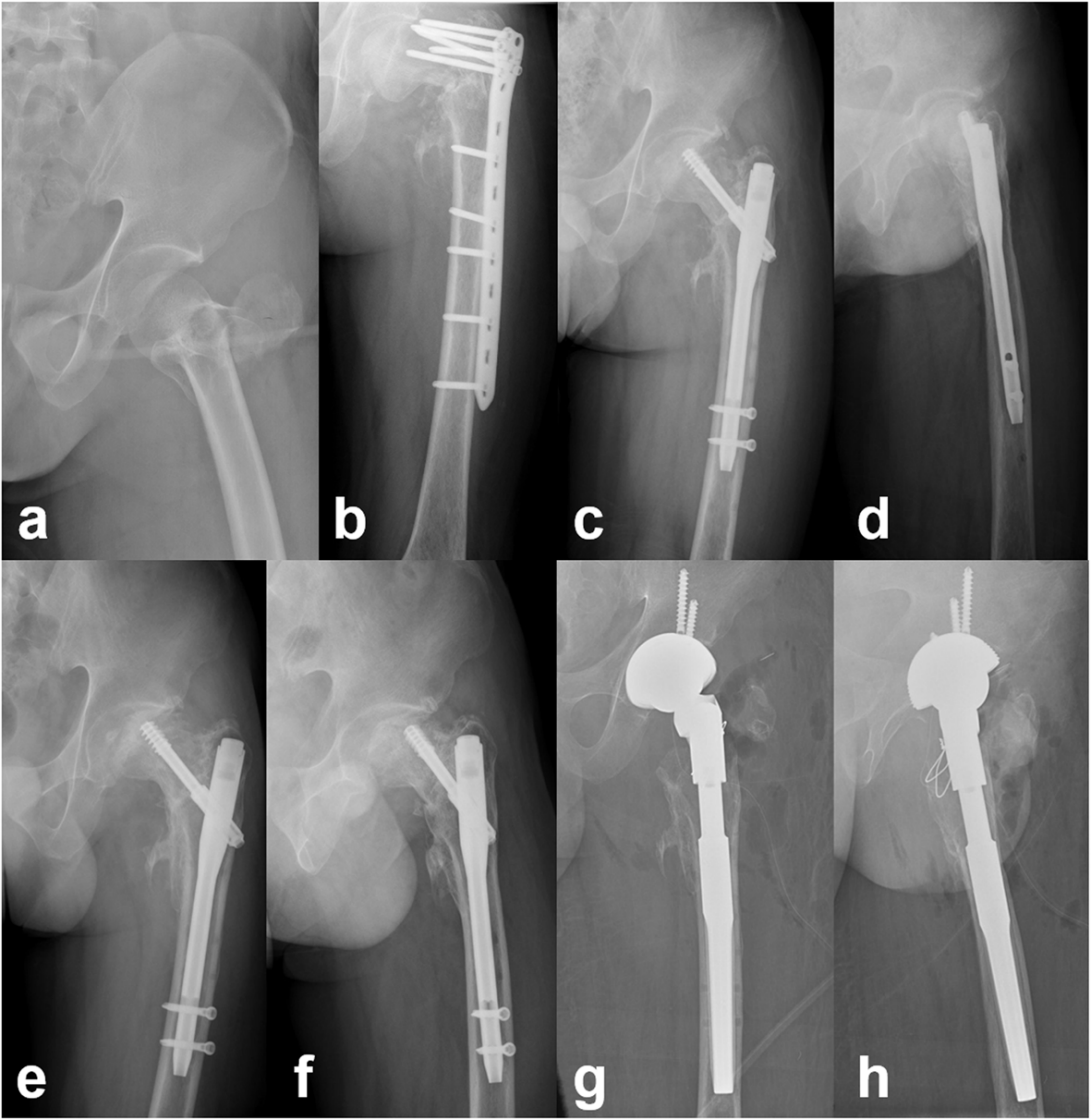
A 42-year-old man with left trochanteric fracture (AO/OTA A3) initially fixed by inverted less invasive stabilization system plate. a The X-ray of original fracture. b Ten months postoperatively, X-ray showed implant failure with varus displacement and nonunion. c-d The previous implant was replaced with a Gamma nail without medial support reconstruction, and the fracture healed nine months after the revision surgery. e–f Twelve months after the revision surgery, X-ray showed avascular necrosis of the femoral head. g-h Twenty-one months after the revision surgery, THA was used as salvage treatment
